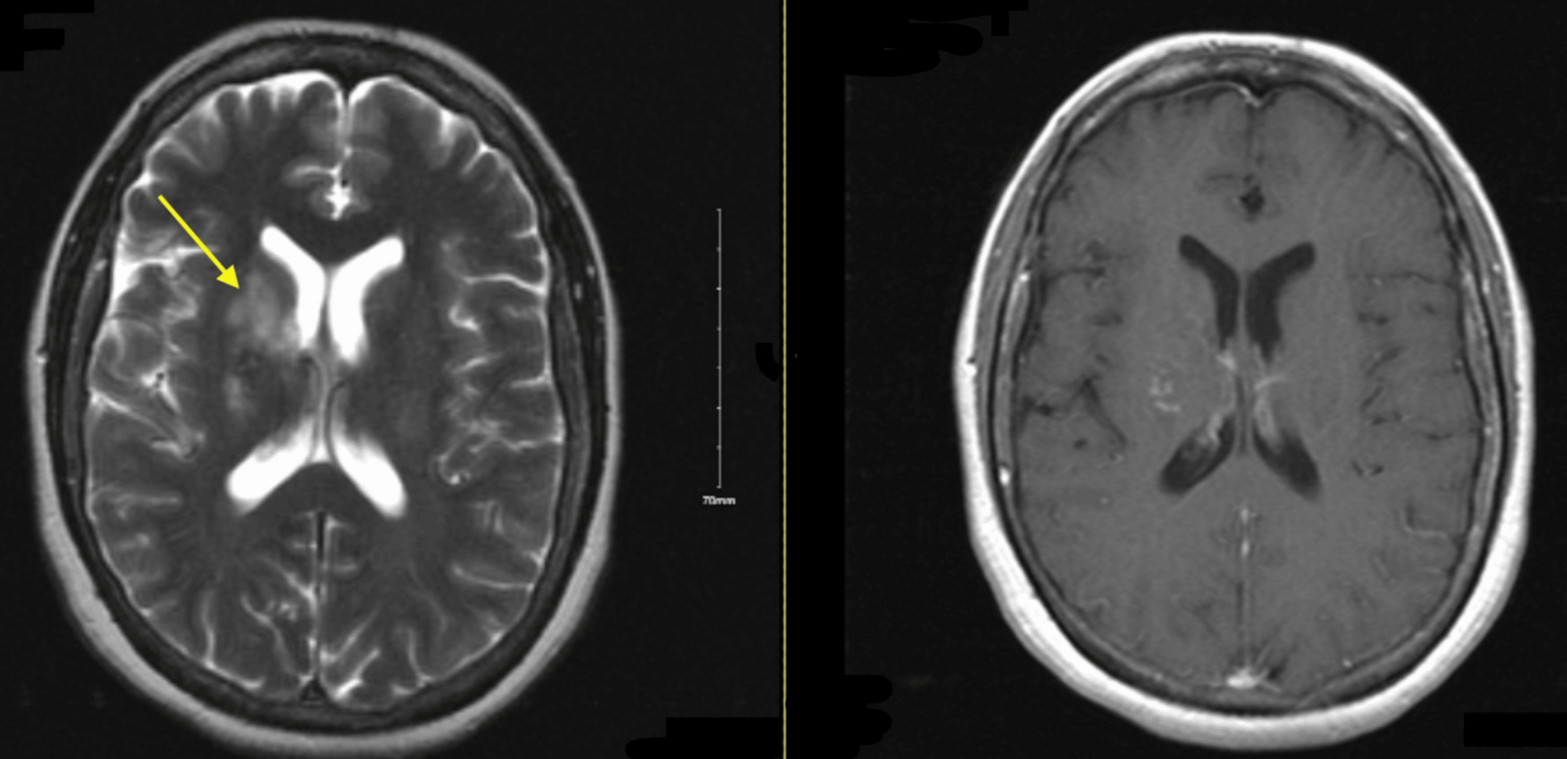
Initial brain MRI and T1 with gadolinium. Left image shows initial brain MRI with and without contrast demonstrating right basal ganglia T2/FLAIR hyperintensity (yellow arrow) extending across the midline to the left, with slight enhancement in the right thalamus and periventricular areas. Right image shows T1 with gadolinium. FLAIR: fluid-attenuated inversion recovery.
